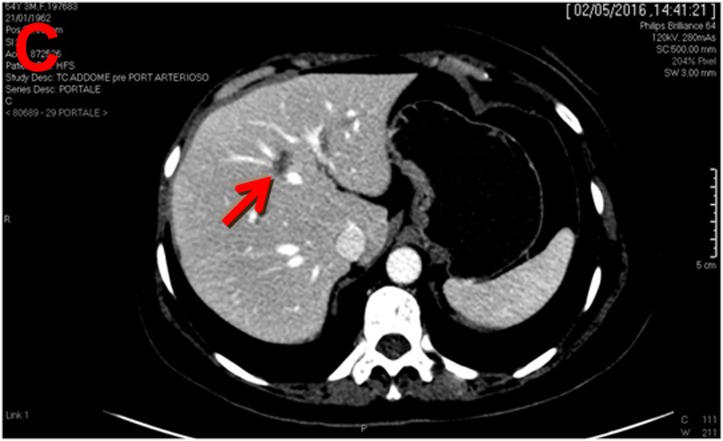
Computed tomography (CT) scan performed on April 2016 shows three liver lesions (LLs) in arterial phase a. The arrow indicates LL (diameter: 7 mm) with contrast enhancement suspected of metastasis at 4^th^ segment; b. The arrow indicates LL (diameter: 19 mm) with contrast enhancement suspected of metastasis at 5^th^ segment; c. The arrow indicates LL (diameter: 18 mm) without contrast enhancement, doubtful lesion for metastasis at 8^th^-4^th^ segment and in differential diagnosis with angioma.
